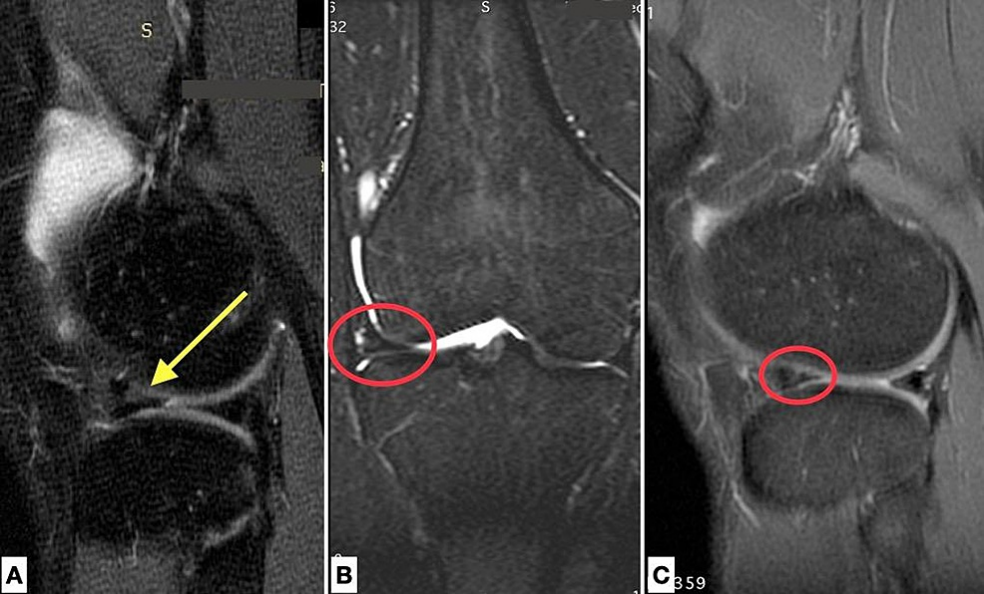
MRI pre-op and post-op. A. Sagittal PD FSE image of the right knee of an 18-year-old male patient with a tear (yellow arrow) in the anterior horn of the lateral meniscus. B. Coronal STIR and C. Sagittal PD FSE image of the same patient 12 months postoperatively, depicting a healed meniscus (red cycle) with signal grade 1.
